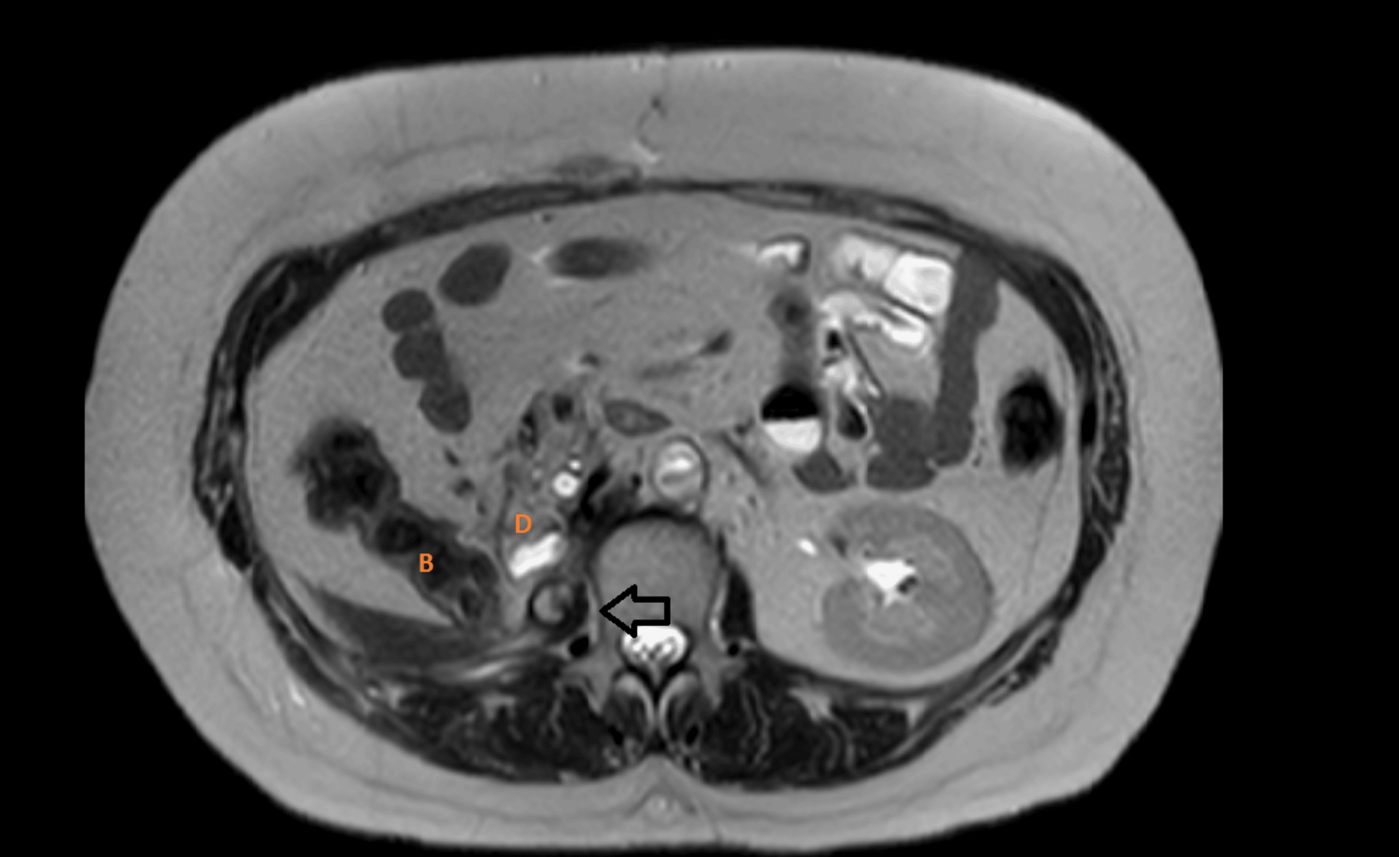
T2-weighted axial MRI image showing a hyperintense lesion (arrow) in the right psoas abutting the duodenum (D) and large bowel (B).
